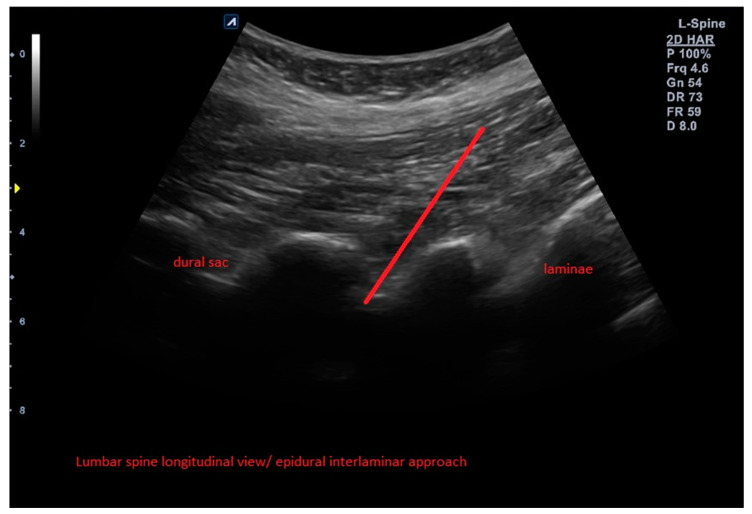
Example of ultrasound-guided epidural ACS injection with needle trajectory in the control group (A).
